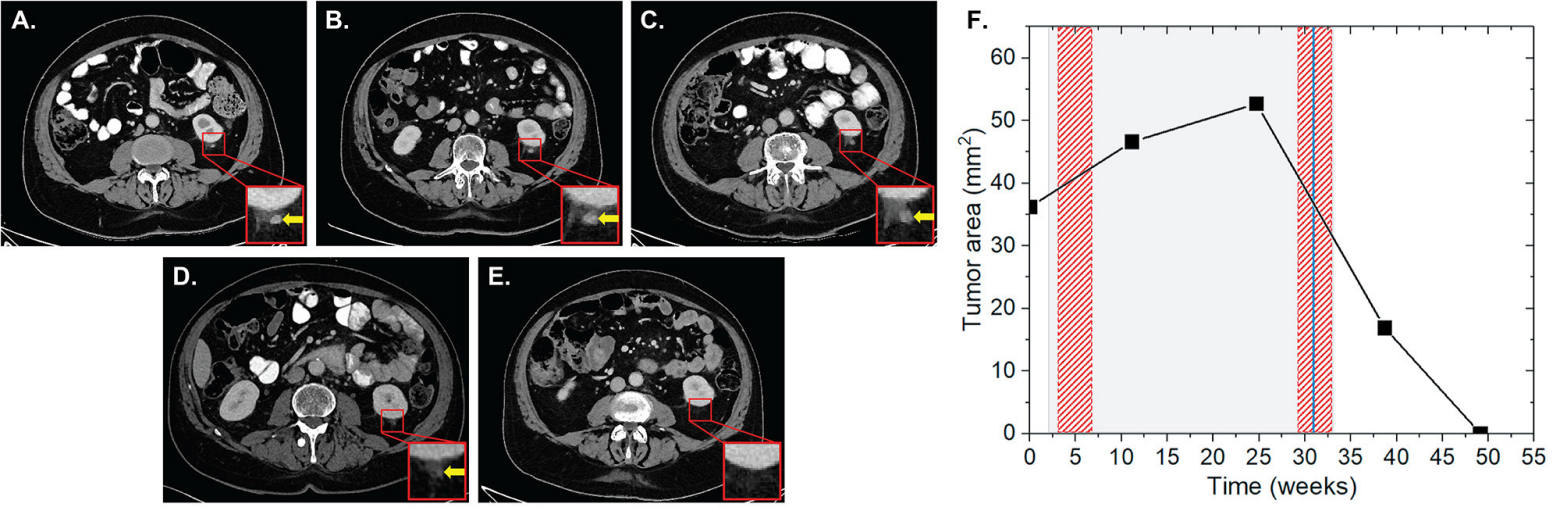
Patient 2 (Table 2B) presenting AbE a soft tissue metastasis in the perirenal region. CT scans show the analyzed lesion (yellow arrows) before (A), and 10 (B), 23 (C), 37 (D), and 47 weeks (E) after the induction of pembrolizumab. (F) The change in the lesion surface area with respect to the administration of IT (duration = 31 weeks, gray shaded area) and concurrent RT (red shaded areas) of the whole brain with 20 × 2 Gy (1 week after 1st IT) and of bone metastases in the left popliteal fossa and lower left leg 18 × 3 Gy (27 weeks after 1st IT) together with SRS of cerebral metastases with 1 × 20 Gy (29 weeks after 1st IT, blue line).
